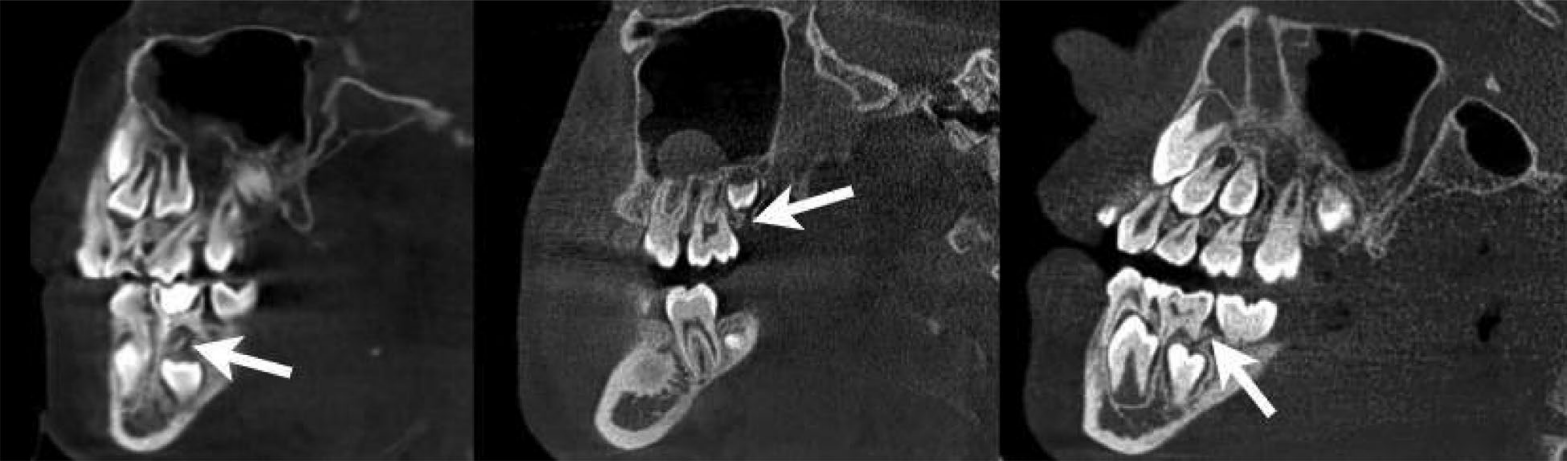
Sagittal image on CBCT of the gubernacular canal (marked by arrow) in posterior teeth
